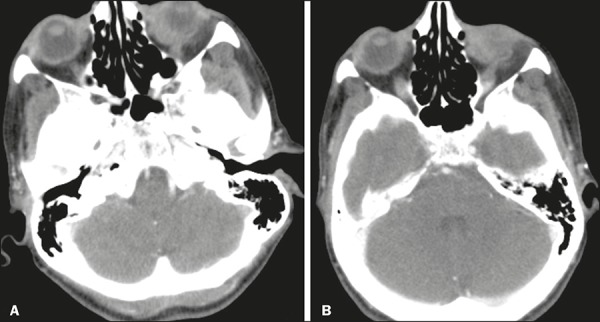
A,B: Contrast-enhanced axial CT scan showing lymphoma of the left ocular
